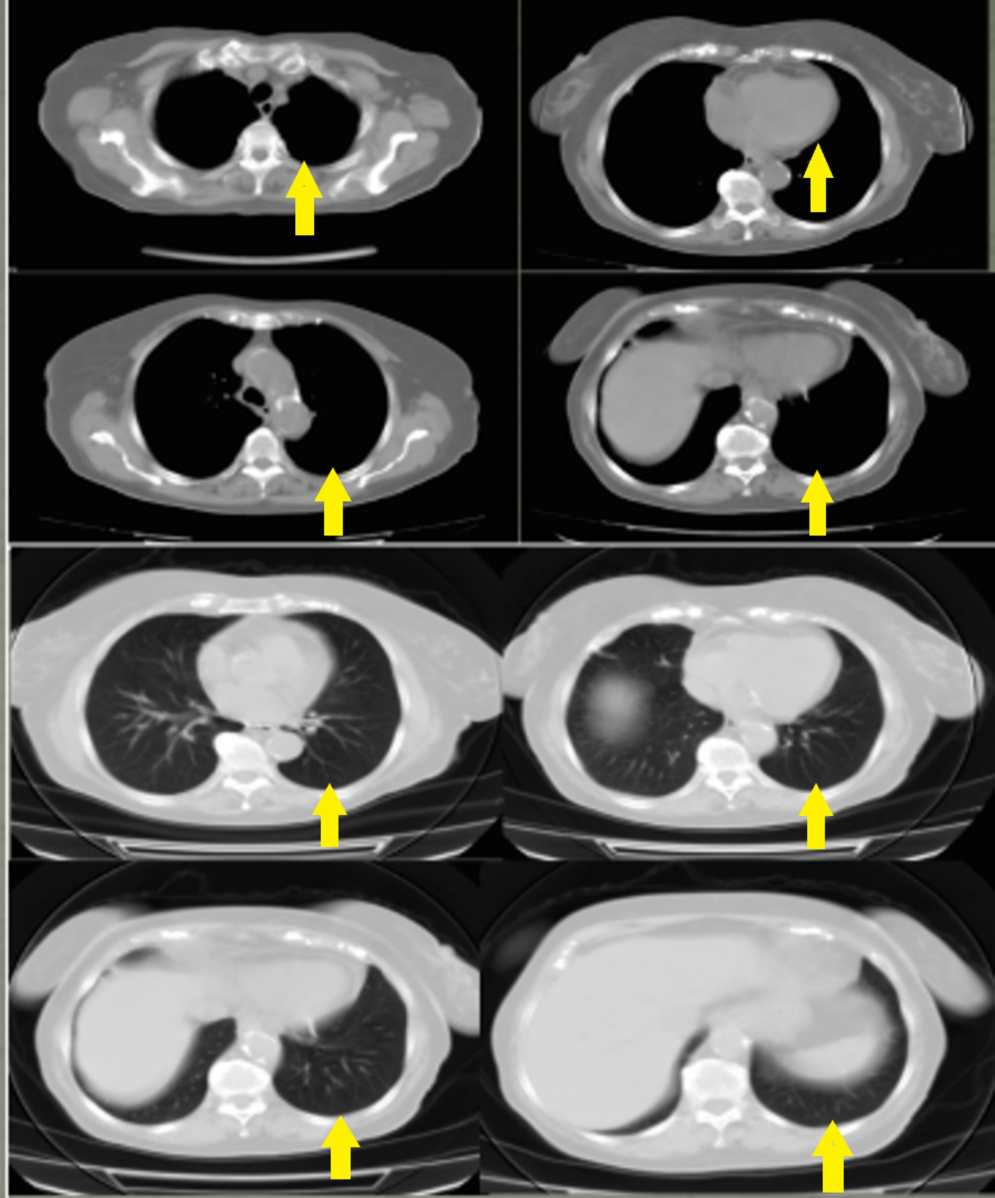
CT of the chest one month after therapy initiation Complete recovery from pleural and pericardial effusions.
